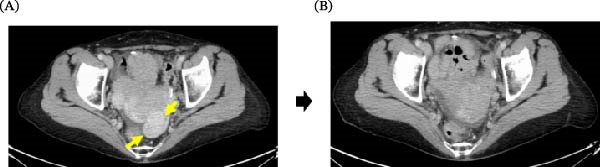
Perioperative computed tomography images of the pelvis in Case 4. (A) The findings 1 month before surgery. (B) The findings 2 months after surgery. The yellow arrow indicates the enlarged left ovary.
